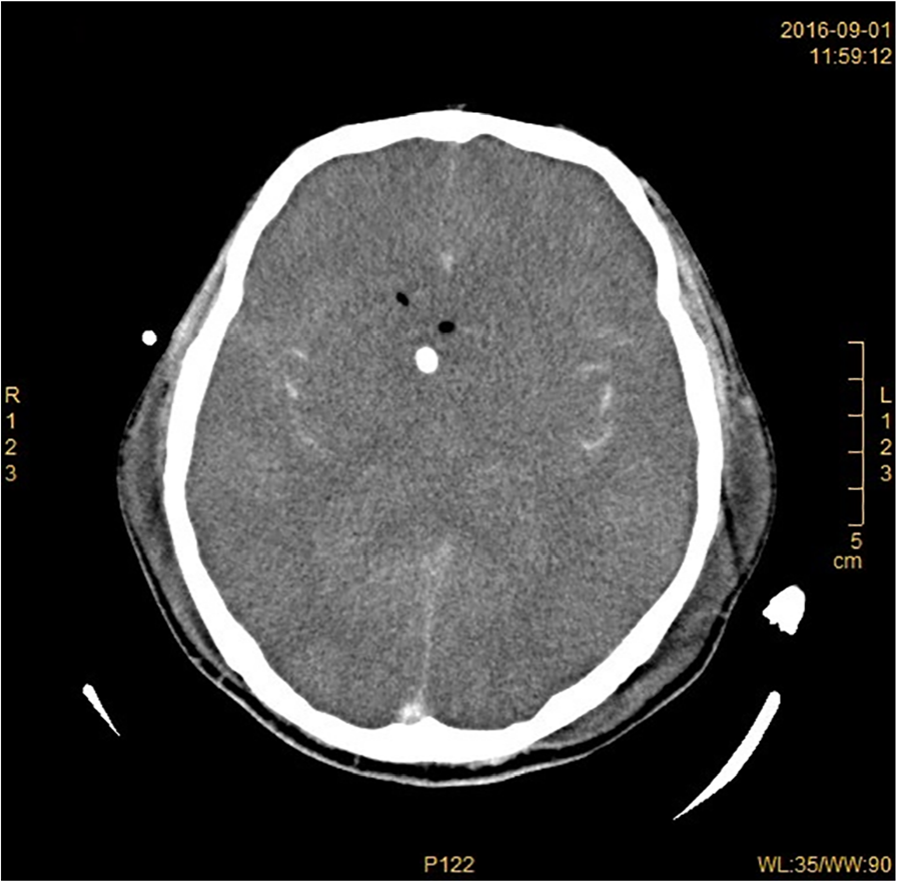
Computed tomography scan of the patient brain with primary amoebic meningoencephalitis
